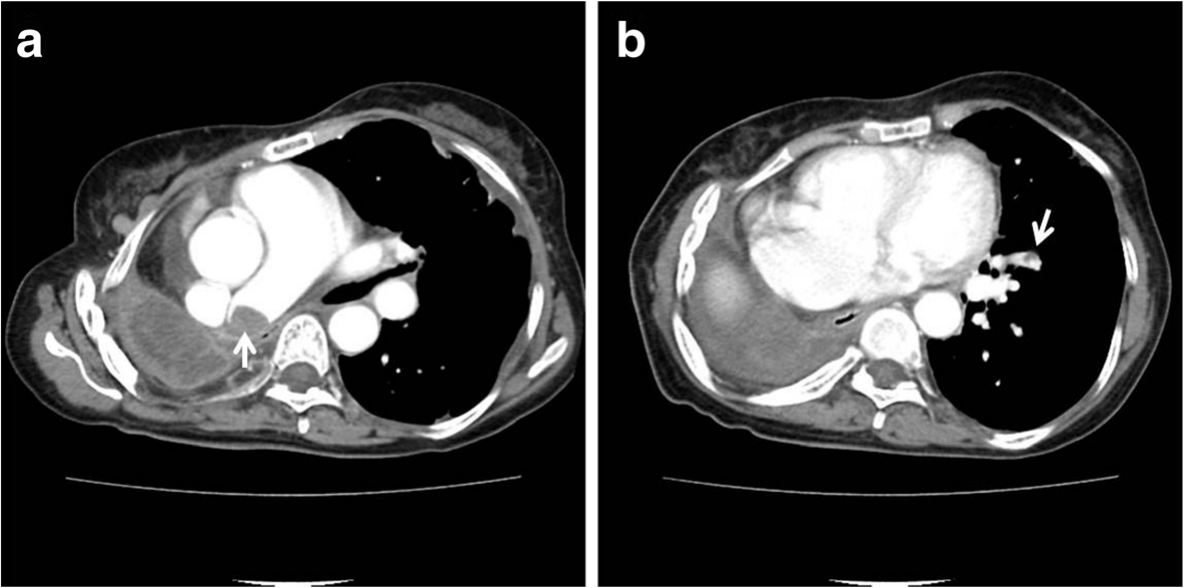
Chest computed tomographic angiography demonstrating about 1.7 × 1.5 cm sized thrombus within the right pulmonary artery stump (a) and pulmonary embolism in left lower segmental pulmonary artery (b)
